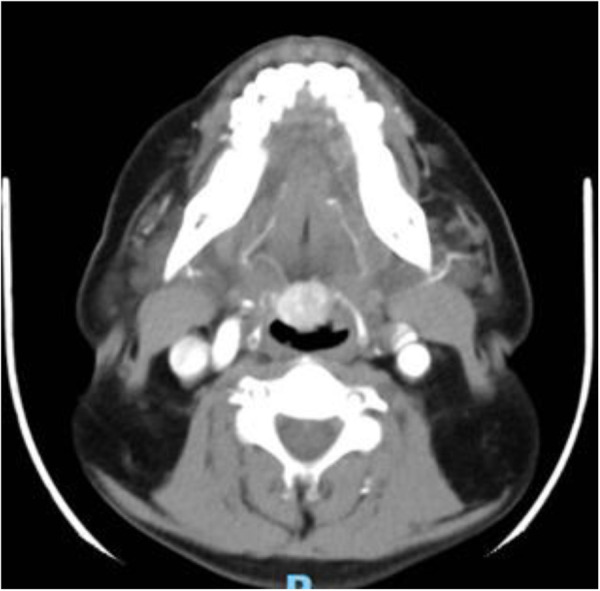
Contrast-enhanced computed tomography findings. Axial view showing an oval hyperdense lesion (1.6 × 1.7 cm) with marked arterial phase enhancement (approximately 85 Hounsfield units) and decreased venous phase enhancement (approximately 45 Hounsfield units), consistent with a characteristic thyroid tissue perfusion pattern.
